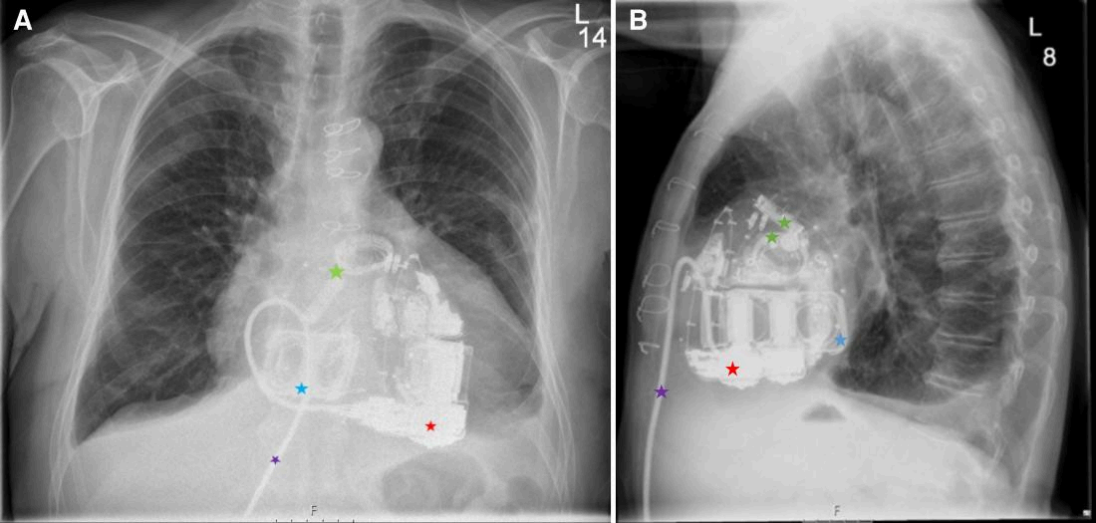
Chest X-ray showing the Aeson® CARMAT Total Artificial Heart, the red star marks the main part of the prosthesis, which contains two micropumps, embedded electronics, and sensors that enable the device to mimic natural heart function through auto-regulated, pulsatile blood flow. The blue star indicates the connection between the atrial flanges and the atrial interface following ventricular removal. The green star marks the pulmonary and aortic valves. The purple star denotes the driveline.
